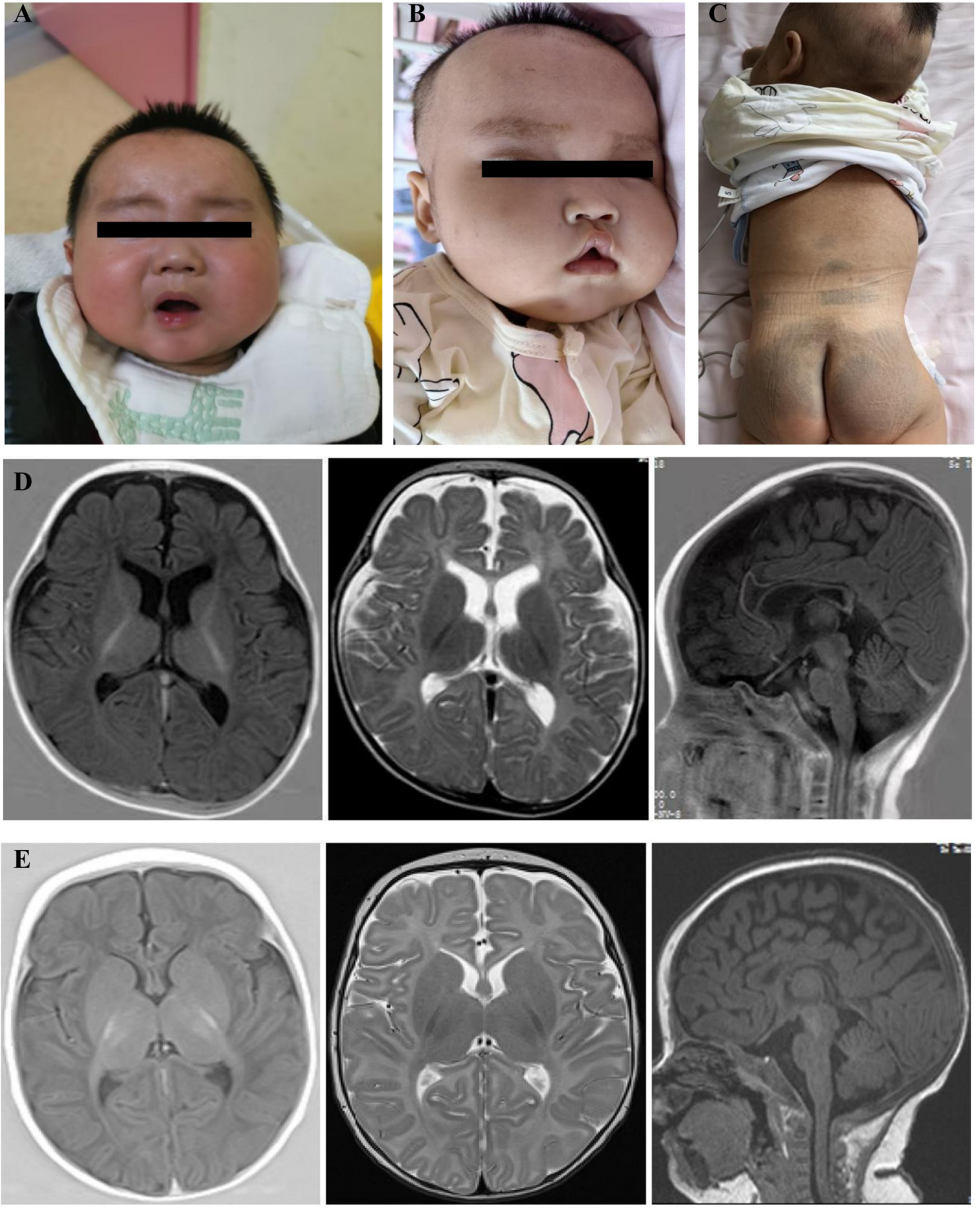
Facial features and brain MRI results of the proband and his younger sister. (A) Facial features of the proband at 19 months of age: thick eyebrows, hypertelorism in both eyes, and collapsed nasal bridge. (B) Facial features of the proband's younger sister at 2 months of age: thick eyebrows, collapsed nasal bridge, tented upper lip, and downward-facing corners of the mouth; (C) Large Mongolian patches on the dorsum and rump of the proband's younger sister (D) Proband, 5-month-old cranial MRI: thin corpus callosum, slightly enlarged lateral ventricles, mildly widened extracerebral spaces in bilateral fronto-temporo-parietal lobes, transverse axial T1W, T2W, and sagittal T1W. (E)Proband's younger sister, 2-month-old cranial MRI: hypoplastic corpus callosum, transverse axial T1W, T2W, and sagittal T1W.
